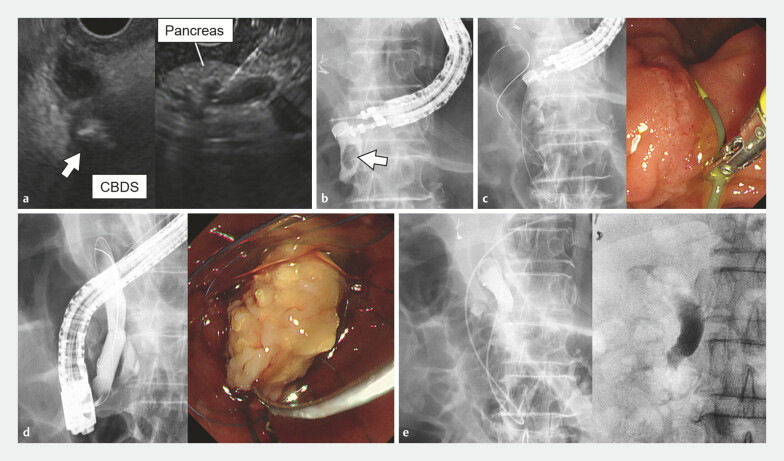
Common bile duct (CBD) stone removal using the endoscopic ultrasound (EUS) rendezvous technique.
